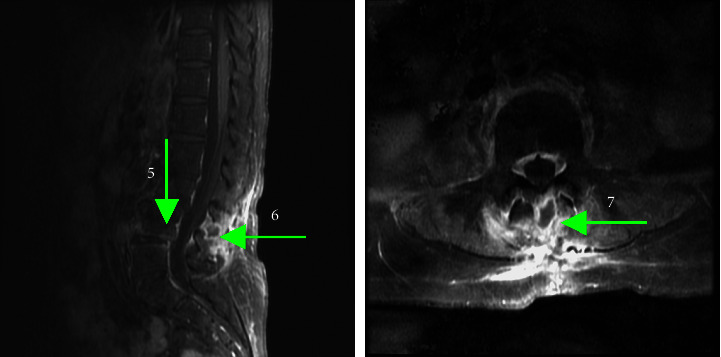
T1 sagittal and axial water-suppression contrast-enhanced MRI obtained approximately 1 month after surgery, showing early stage of infective spondylodiscitis between L4 and L5 (arrow 5) and Baastrup's disease between L3 and L4 with secondary abscess transformation (arrows 6 and 7).
